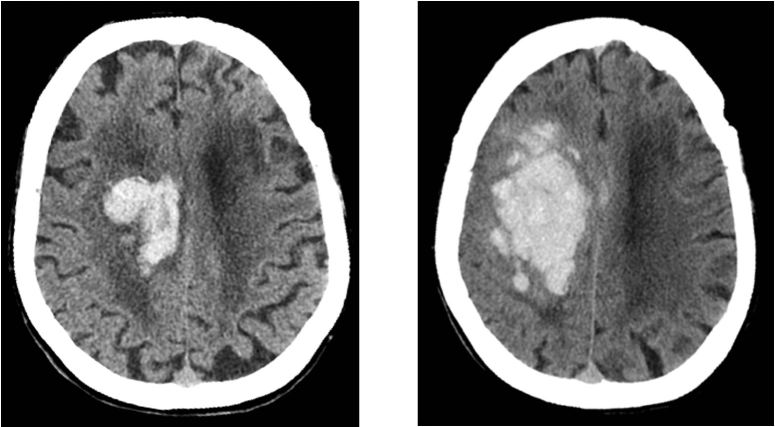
Noncontrast head computed tomography performed (top left) at initial presentation and (top right) six hours following injury demonstrates interval progression of the intraparenchymal and intraventricular hemorrhage centered predominately in the region of the right centrum semiovale, as well as corona radiata and body of the right lateral ventricle. This patient was on a coumadin and aspirin combination therapy with a presentation Glasgow Coma Scale of 13 and uneven pupillary response on neurological examination. This patient expired during the hospitalization.
